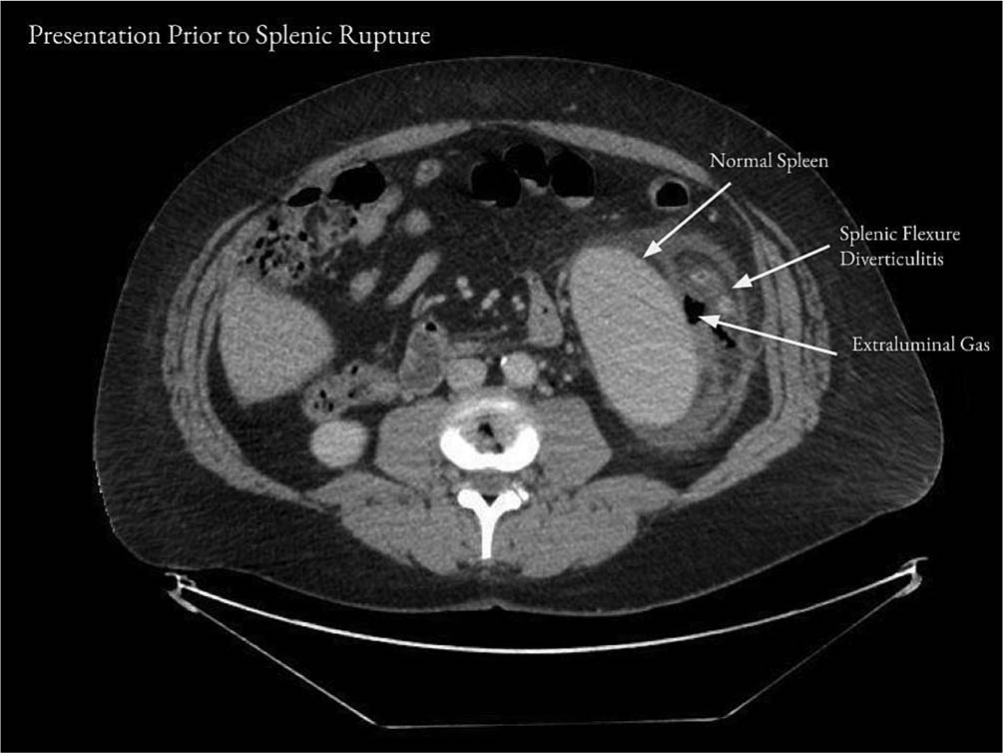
Computed tomography of the abdomen and pelvis on presentation showed acute diverticulitis of the splenic flexure with moderate wall thickening and adjacent extraluminal gas consistent with localized perforation.
